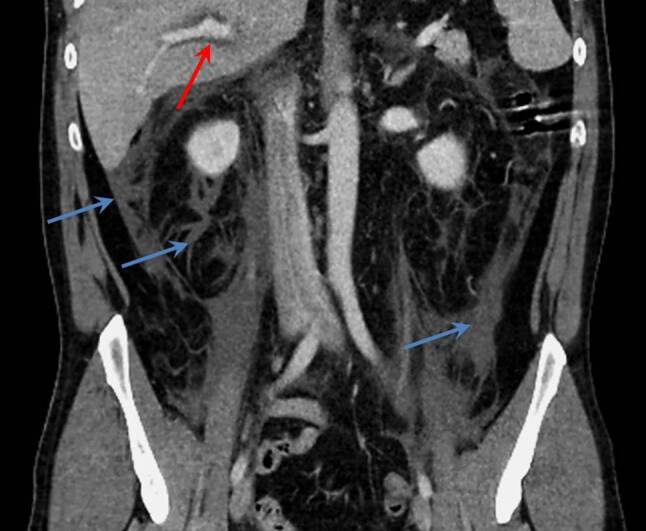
Contrast-enhanced abdominal CT scan in the coronal plane demonstrating mildly dilated intrahepatic bile ducts (*red arrow*) and retroperitoneal fluid collections (*blue arrows*)
